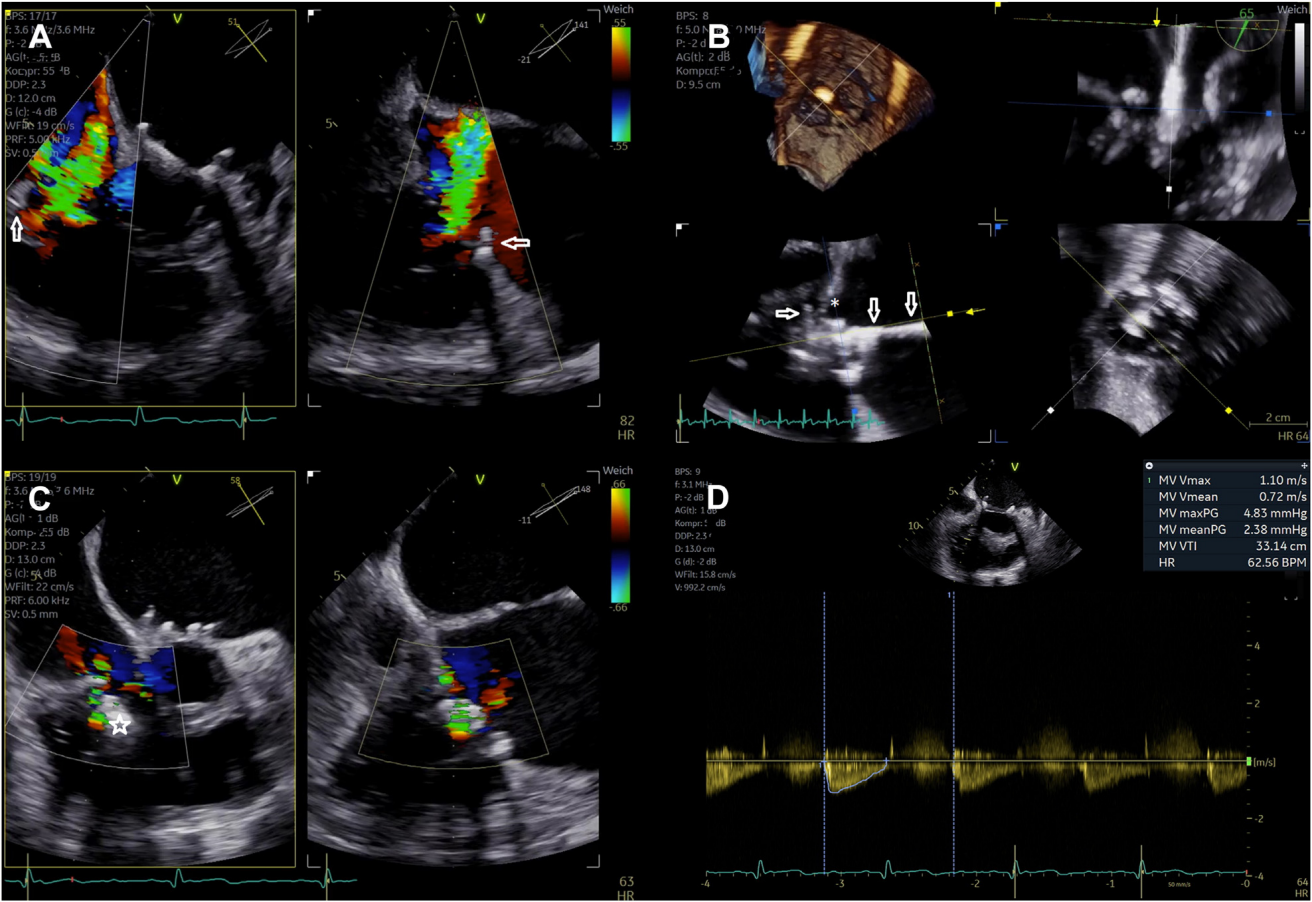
Periprocedural Transesophageal Echocardiographic Guidance (A) Severity of tricuspid regurgitation before performing tricuspid transcatheter edge-to-edge repair (arrows mark the previously implanted annuloplasty ring). (B) Multiplanar reconstruction to visualize the septal leaflet with the delivery system already positioned subvalvularly (arrows, delivery system; asterisk, septal leaflet). Residual tricuspid regurgitation is seen in C (star, transcatheter edge-to-edge-repair device), and (D) confirms adequate postprocedural transvalvular gradient of the tricuspid valve.
